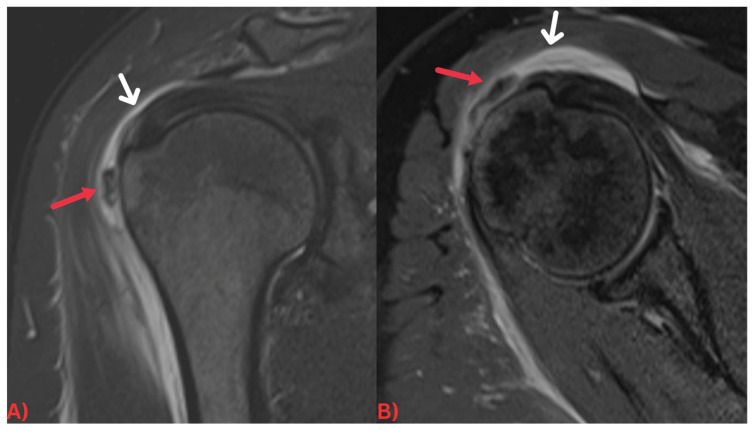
(A) Coronal PD fat-saturated MRI and (B) axial PD fat-saturated MRI images of the right shoulder demonstrating a few ill-defined low signal intensity calcifications (red arrows) in the subacromial/subdeltoid bursa, with fluid signal intensity within the bursa (white arrows) most suggestive of calcific bursitis.
